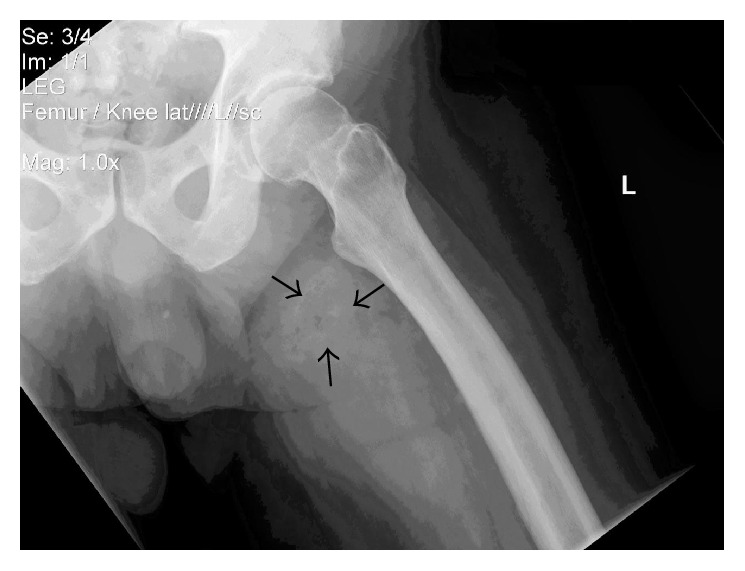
Radiograph of left leg demonstrating gas within the necrotic wound (arrows).
